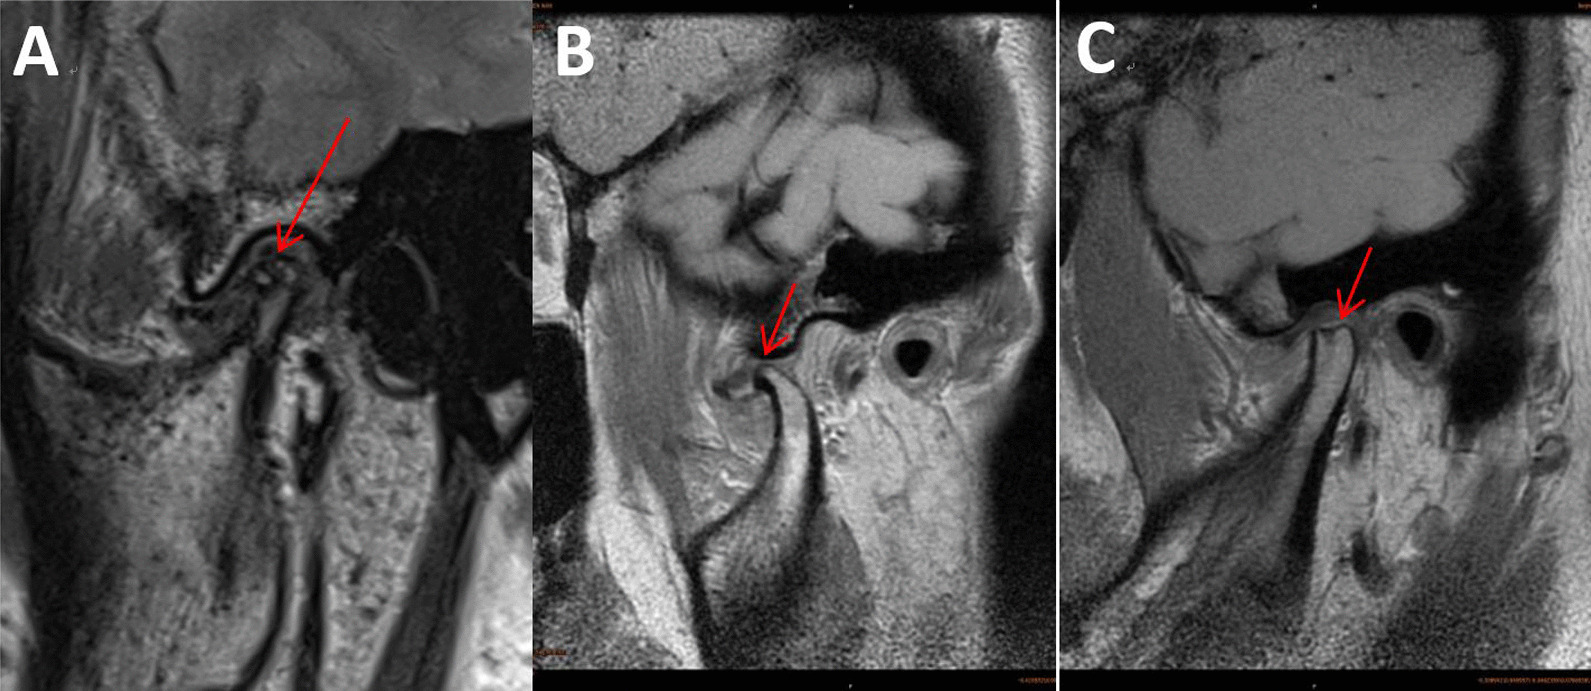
Sagittal MRI of TMJ. A Osteophytosis. B Erosion and sclerosis. C Mandibular condylar erosion and joint eminence
